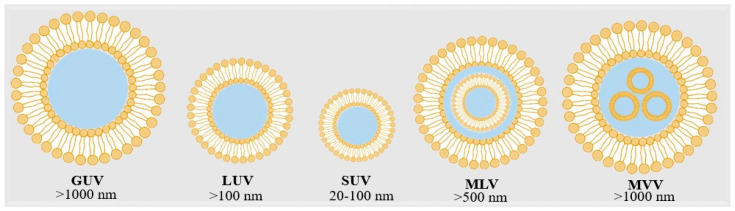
Types of liposomes based on lamellarity: small unilamellar vesicle (SUV), large unilamellar vesicle (LUV), multilamellar vesicle (MLV), multivesicular vesicle (MVV), and giant unilamellar vesicle (GUV).
